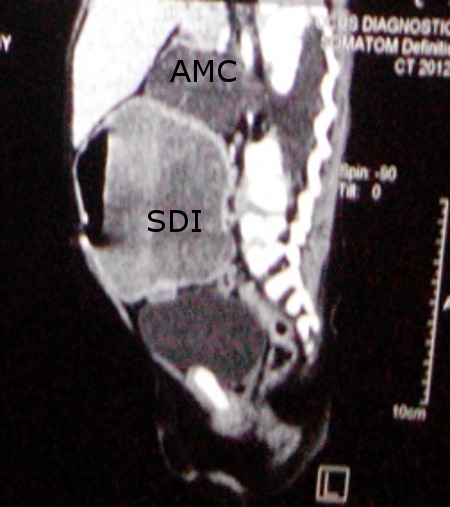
Figure 1: MRI showing SDI and AMC.
